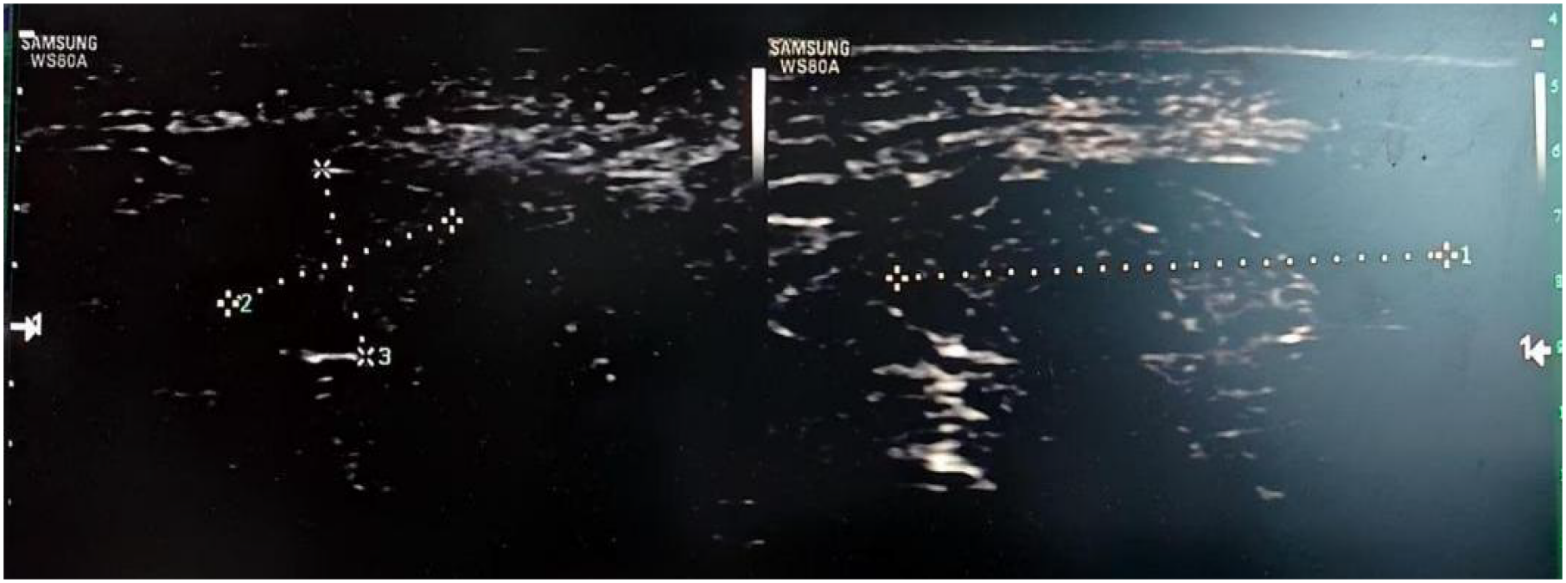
Preoperative transvaginal gynecologic ultrasound of the patient revealed that the right vulvar “mass” measured about 2.0*0.9*0.7 cm.
